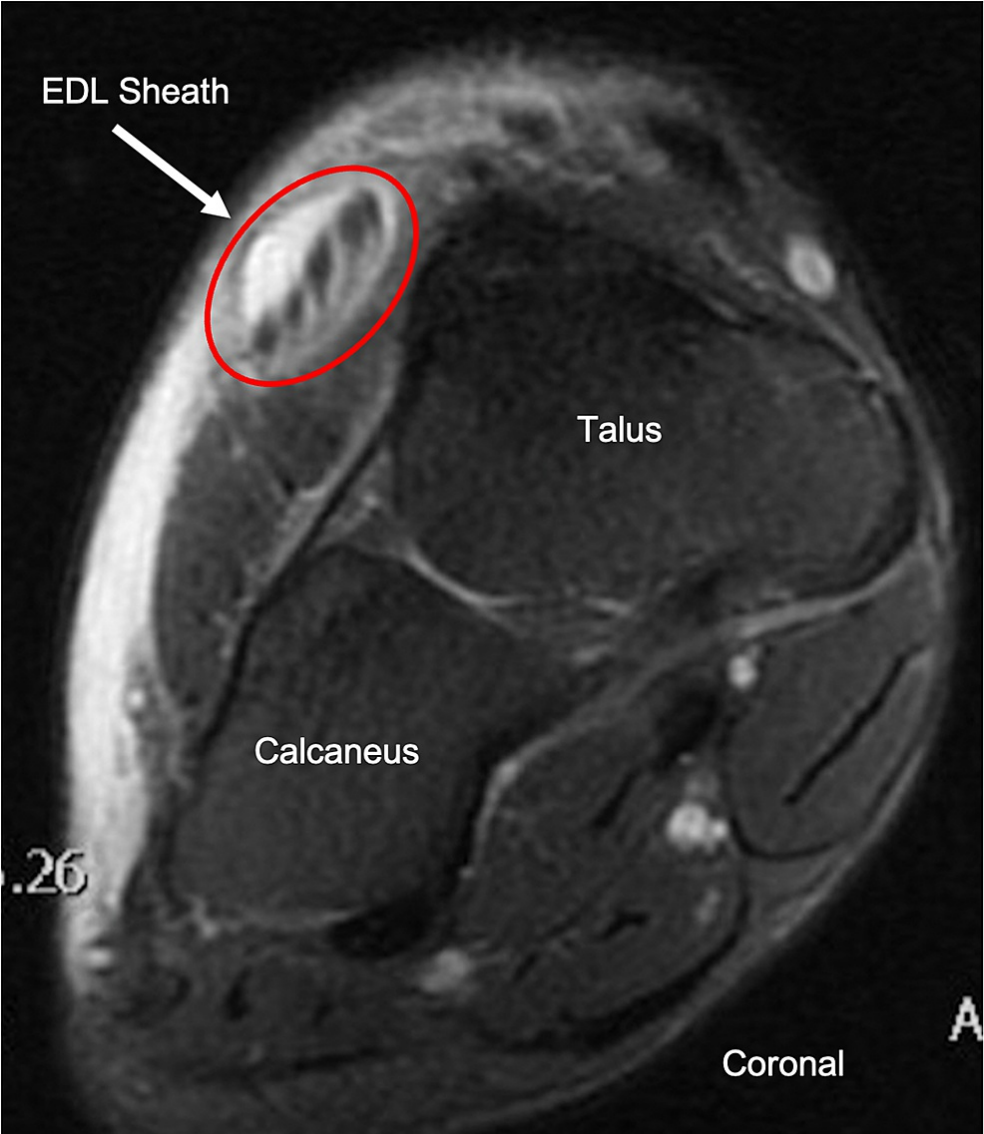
Coronal T2 FS MRI with IV gadolinium of the right foot showing dorsal soft tissue swelling and fluid within the extensor digitorum longus tendon sheath FS = fat-saturated, MRI = magnetic resonance imaging, IV = intravenous, EDL = extensor digitorum longus
